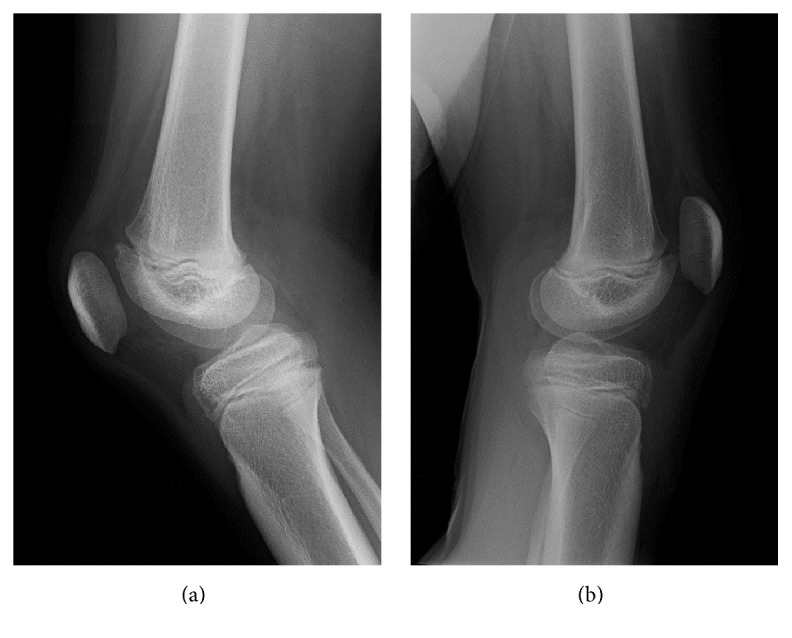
Preoperative lateral radiographs of the (a) right knee and (b) left knee.
